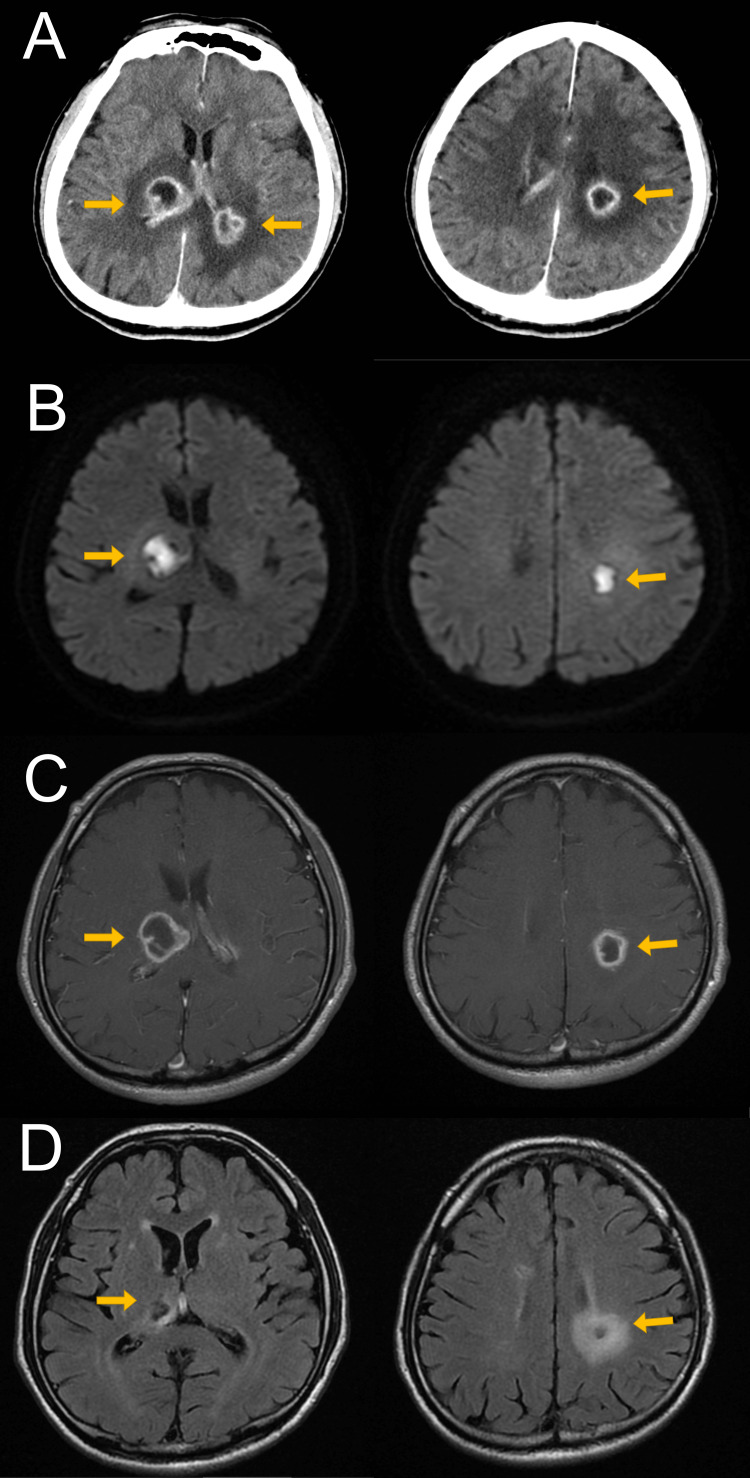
Brain computed tomography and magnetic resonance imaging (A) Contrast-enhanced CT of the brain at the time of admission shows the ring-enhanced low-density area (arrows). (B) Diffusion-weighted MRI of the brain at the time of admission shows a marked high-signal area (arrows). (C) Contrast-enhanced MRI images of the brain at the time of admission show a uniform high-signal capsule and an internal low-signal area (arrows). (D) Contrast-enhanced MRI images of the brain at the time of discharge show a size reduction in both brain abscesses (arrows).
